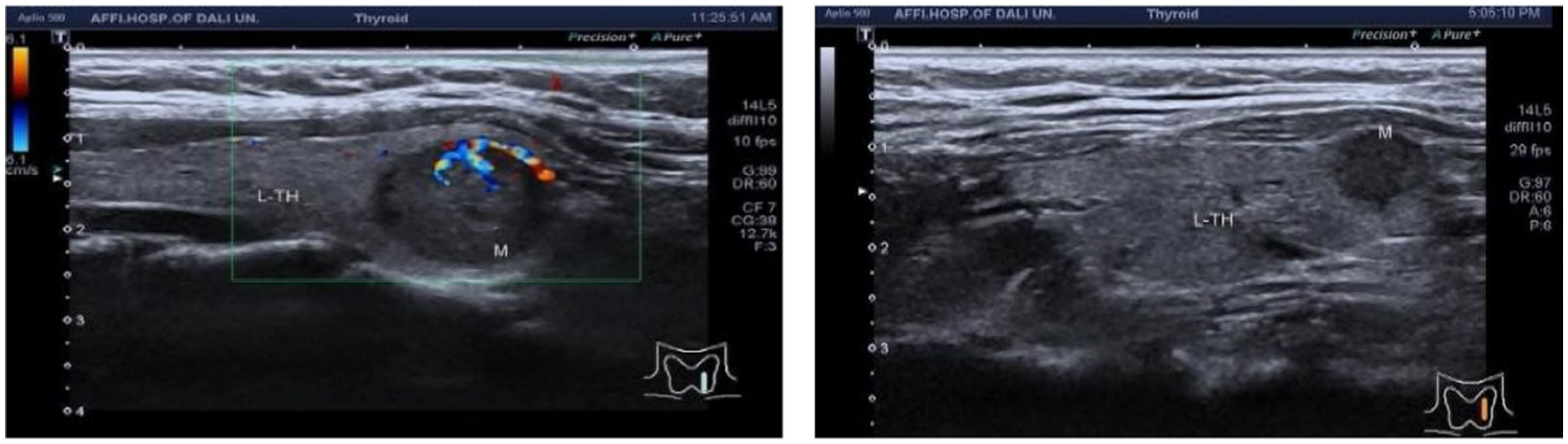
Ultrasound image of nodular lesion in the left lobe of the thyroid gland, C-TIRADS classification: 4A.
